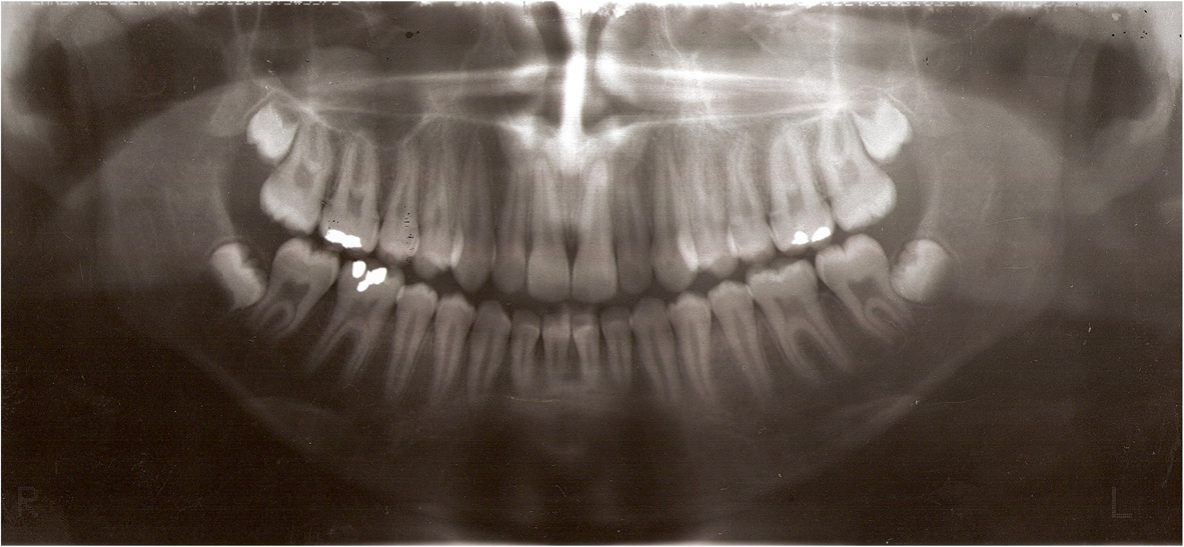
Panoramic radiograph at T0
